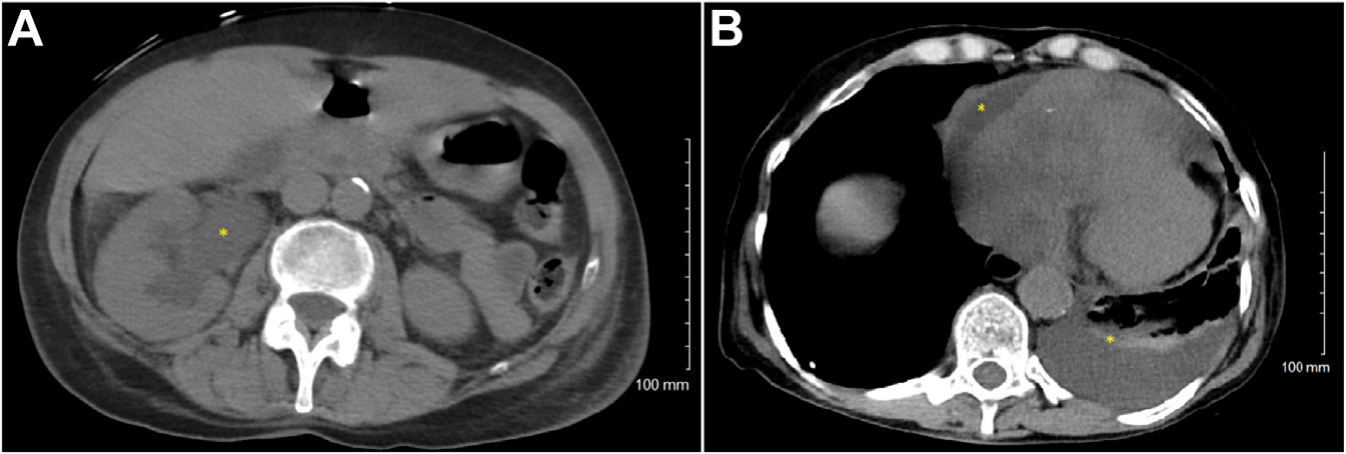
Computed Tomography of the Abdomen (A)The right-sided hydronephrosis and mass at the renal pelvis suggestive of malignancy. (B) Neoplastic changes in the mediastinum, pericardial thickening, and a left lower lobe pleural effusion that appears exudative and is suggestive of a metastatic process.
